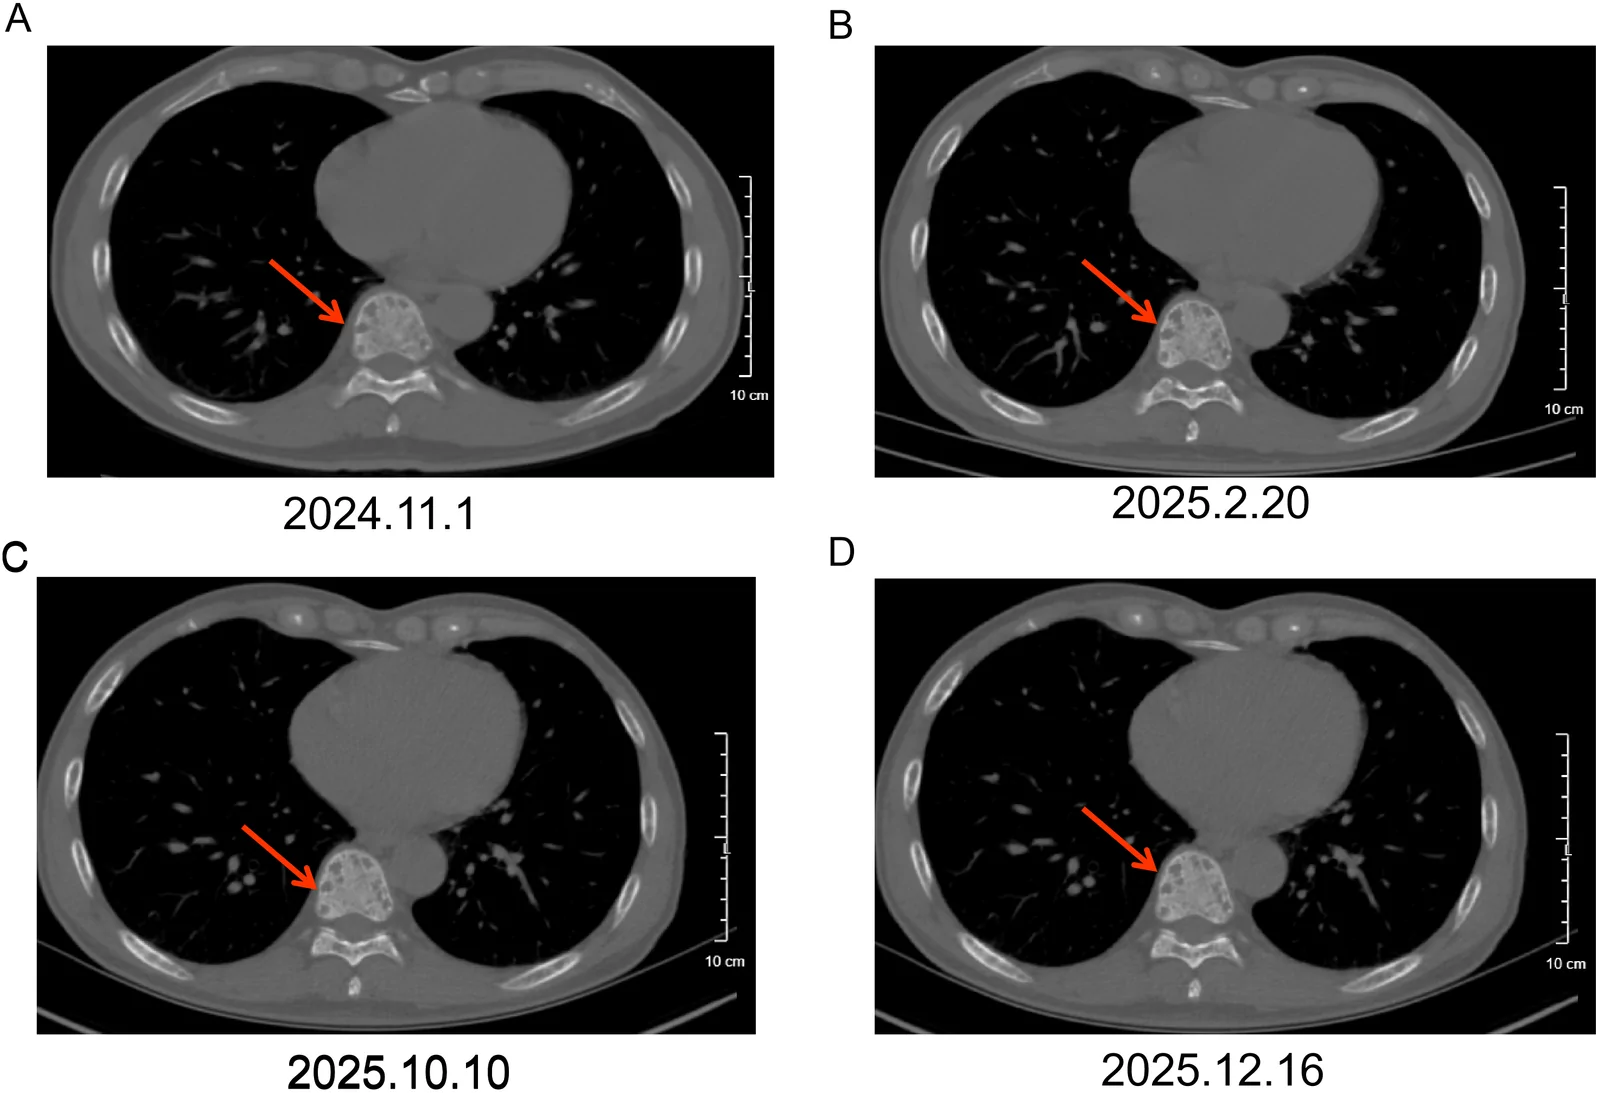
A series of chest CT scans were recorded osteolytic lesions of the thoracic vertebrae that maintained stable conditions (A-D).
